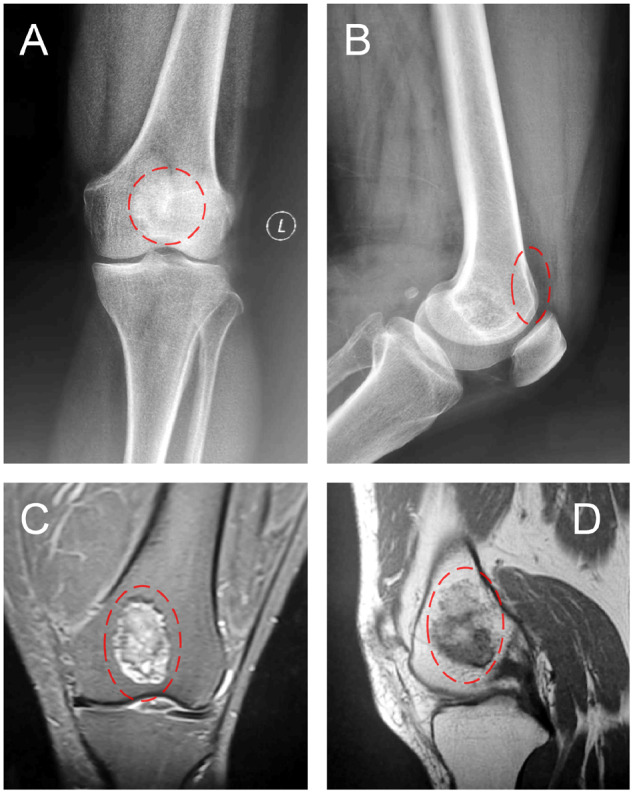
Preoperative imageological examinations of distal femoral space-occupying lesion of the 45-year-old female patient (A, frontal view of plain radiography; B, lateral view of plain radiography; C, coronal MRI; D, sagittal MRI; the lesion is noted by dashed circle in each picture).
